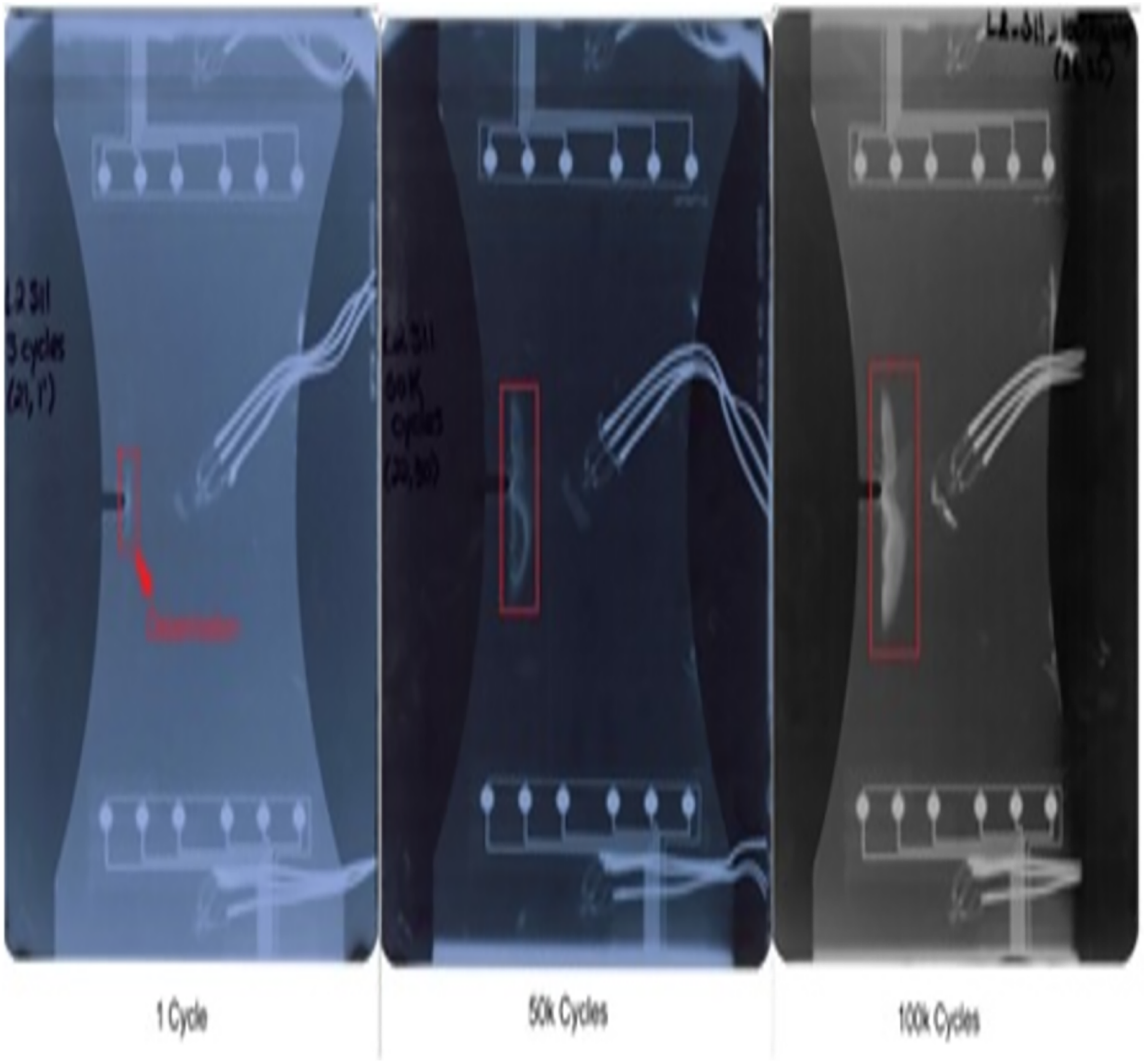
X-ray images for various loading cycles.
